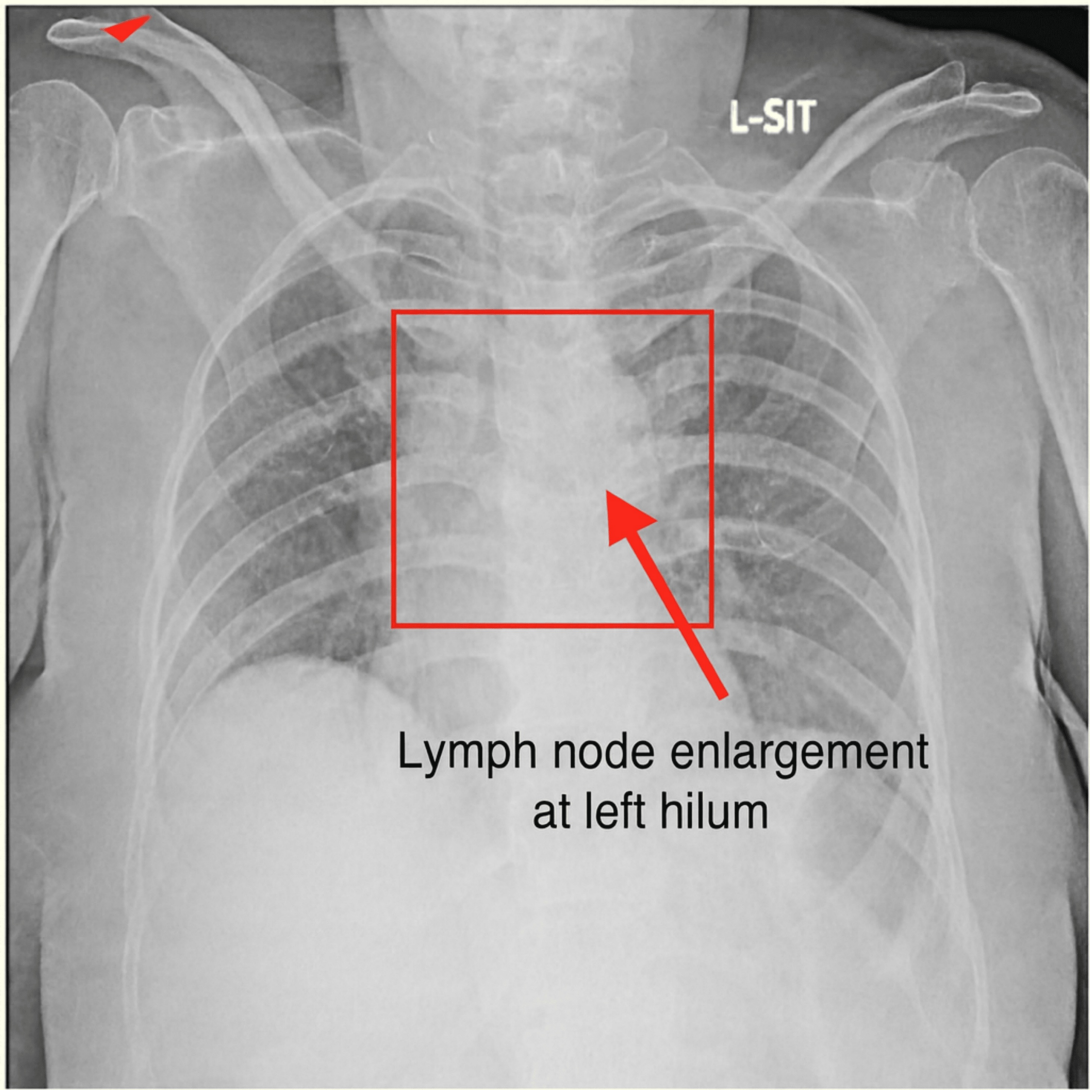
Chest X-ray (posteroanterior view) showing lymph node enlargement at the left hilum (red arrow).
